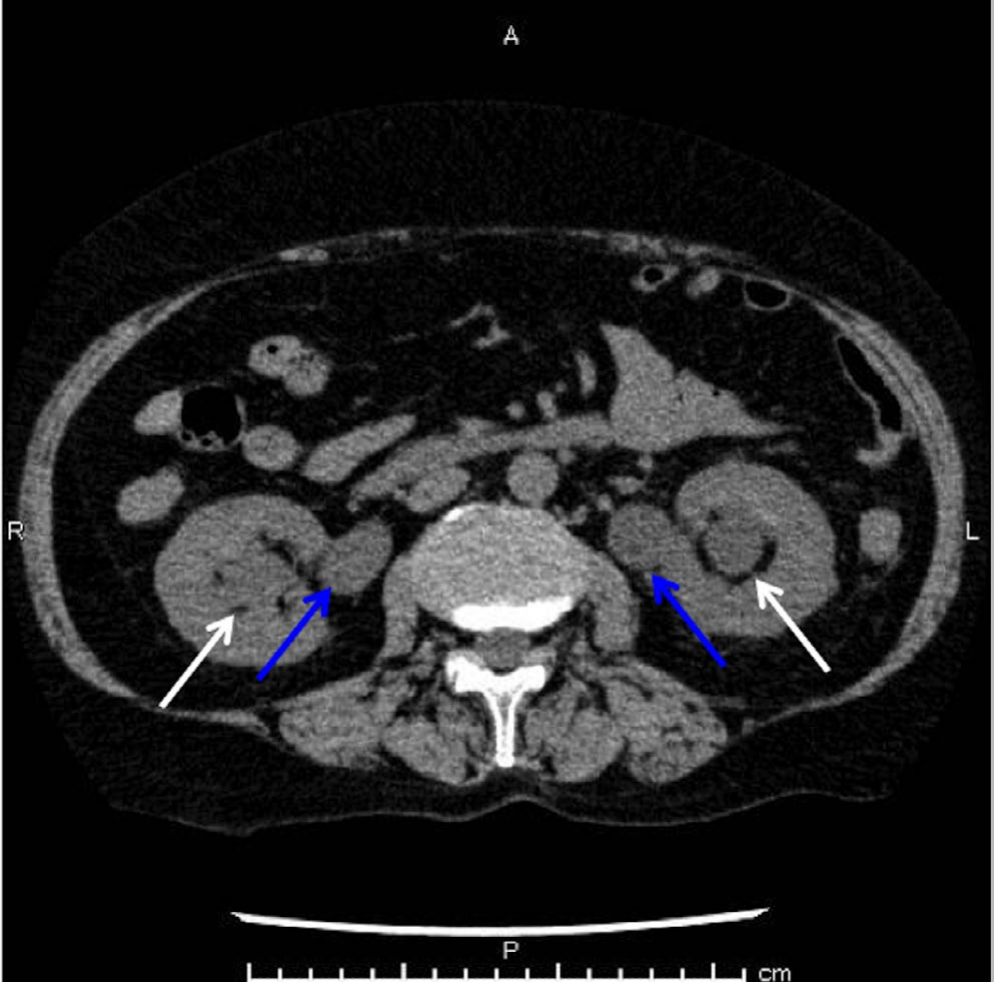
Computed tomography (CT) imaging findings. Transverse view shows bilateral hydroureteronephrosis (white arrow) and hydroureter (blue arrow)
